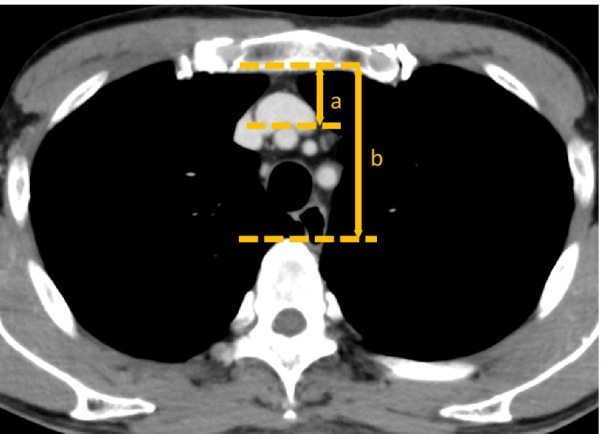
The width of retrosternal space was defined by the ratio of a to b. a the back of the sternum to the ventral part of the brachiocephalic artery. b the back of the sternum to the ventral part of the vertebra. To evaluate the retrosternal space, Contrast-enhanced CT was conducted preoperatively within 3 months
